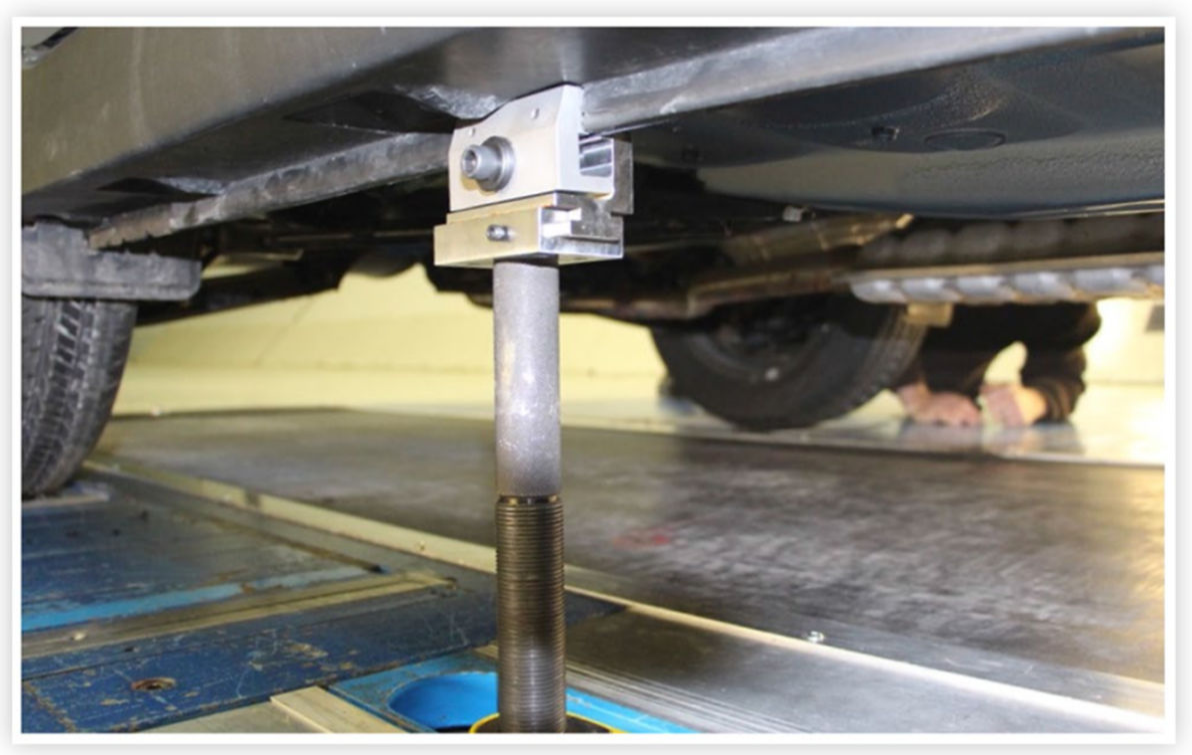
Close-up view of a rear GESS strut and rear GESS clamp attached to the rocker panel of a small SUV.
